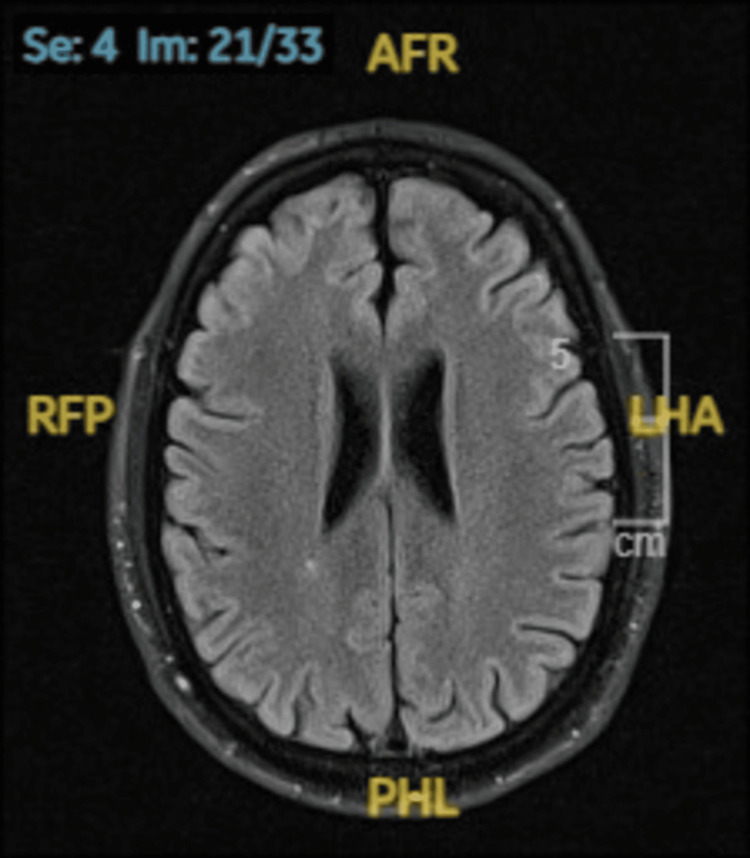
Axial brain FLAIR MRI sequence demonstrating normal cerebral parenchyma with no evidence of acute infarction or focal pathology Image obtained from the hospital radiology PACS and anonymised for publication FLAIR: fluid-attenuated inversion recovery, MRI: magnetic resonance imaging, PACS: Picture Archiving and Communication System
